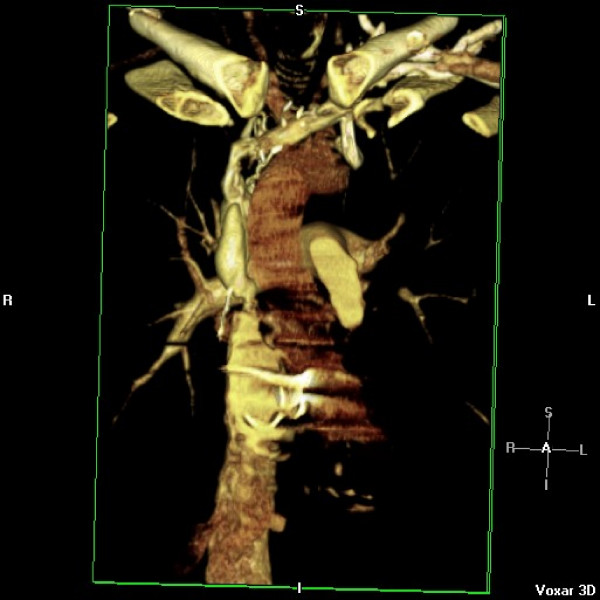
Contrast CT angiogram showing residual stenosis both at the confluence of the two innominate veins and at the junction of SVC with right atrium.
